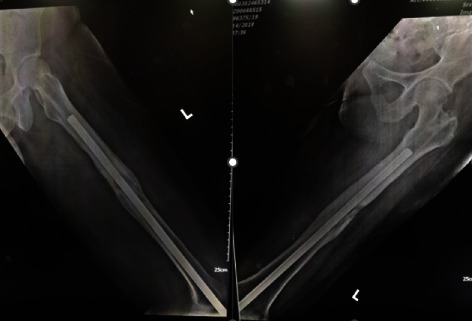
Plain radiograph shows the feature of chronic osteomyelitis over femur shaft.
